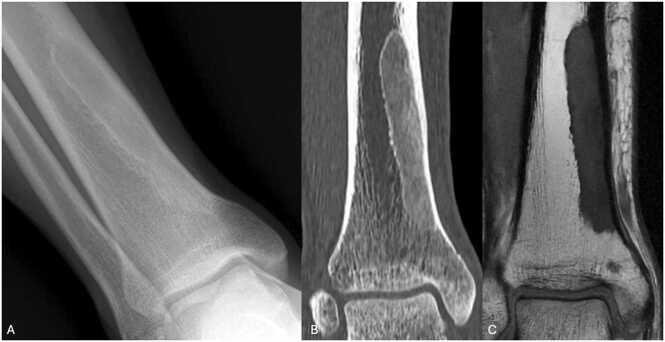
Fibrous dysplasia in the distal third of right tibia in a 32-year-old man presenting pain. A. Face radiography showing a large oval ground-glass lesion with sclerotic margins of the distal third of right tibia. B, CT image in the coronal plane highlighting the aspect of ground-glass. C. T1-weighted MR image in the coronal plane showing a homogenous hyposignal with hyposignal corona (white arrow).
